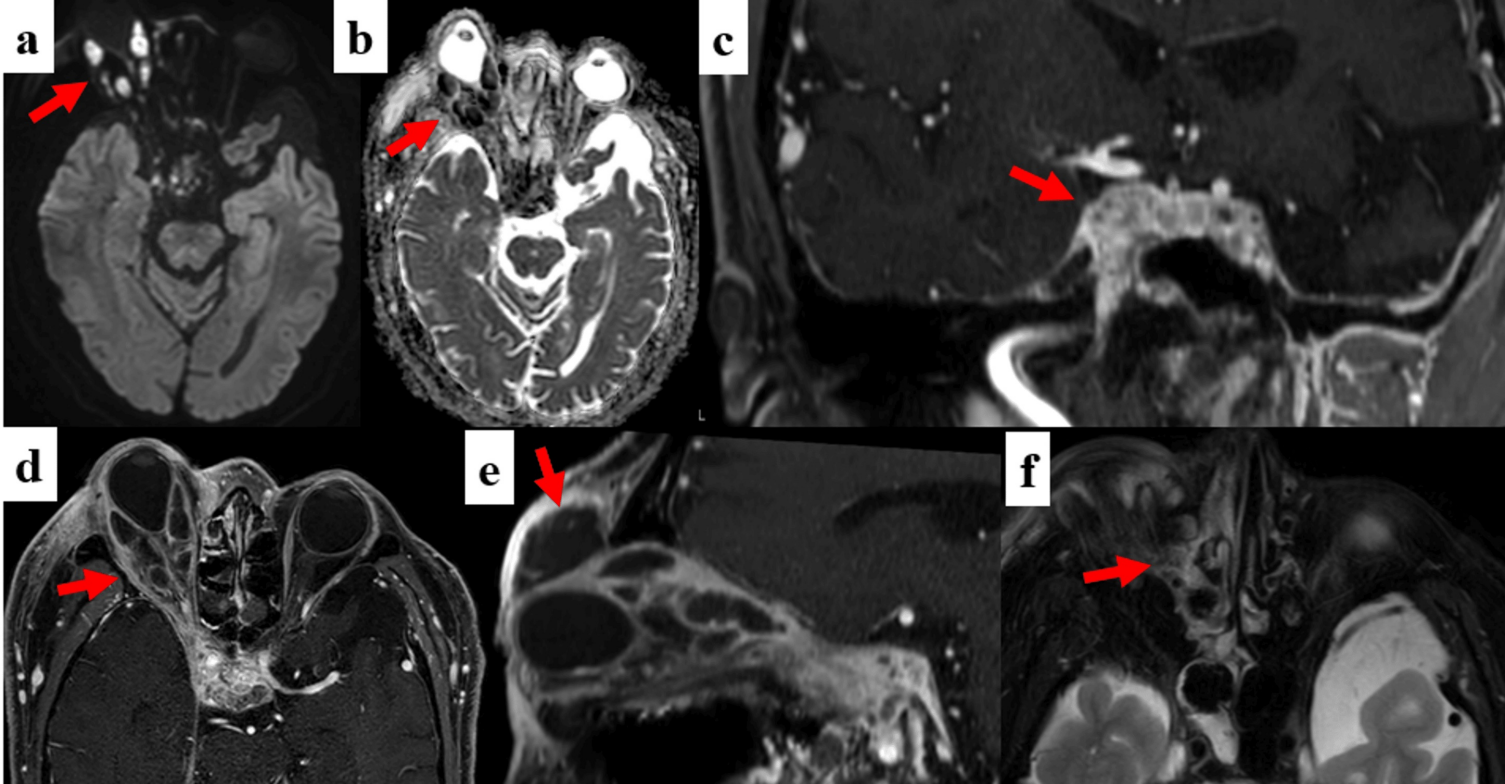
Orbital MRI on admission (a, b) Abscesses in extraocular muscles showed hyperintensity in diffusion-weighted imaging (DWI) with apparent diffusion coefficient (ADC) reduction (arrow). (c, d) Inflammation spread towards the right orbital apex and cavernous sinus (arrow), which showed prominent gadolinium enhancement. (e) There was also abscess formation in the right upper eyelid, where incisional drainage and sampling were done (arrow). (f) Fluid accumulation was seen in the right sphenoid sinus (arrow), suggesting sinusitis as one of the infection routes.
